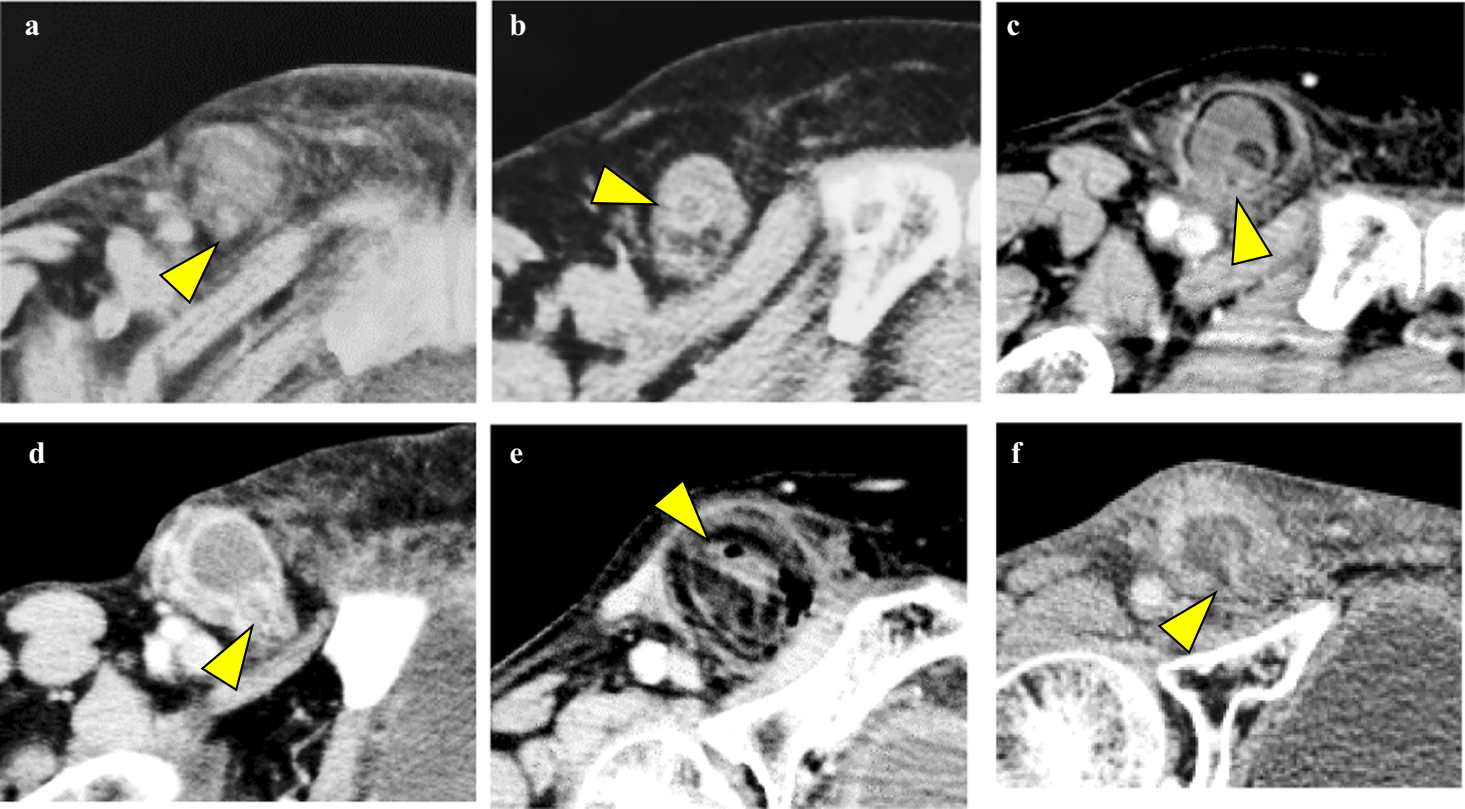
Axial images of contrast enhancement CT showing a tubular structure (arrowhead) surrounded by or along with high/iso/low-density masses on the ventral and medial sides of the femoral vein in Cases 1–6 (a–f in this order). d, f: A low-density ovoid lesion with high-density capsule suggestive of an abscess around the appendix. e: A honeycomb-like low-density mass surrounding a tubular structure suggestive of the greater omentum
